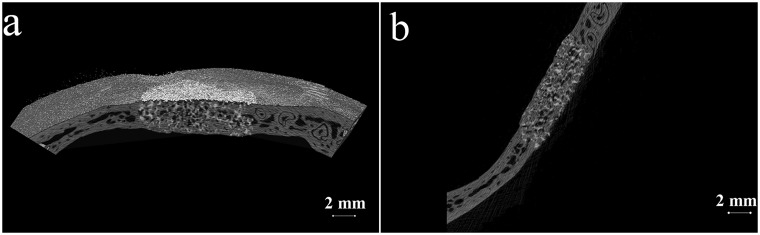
The 3D (a) and 2D (b) reconstructed images by μ-CT analysis
